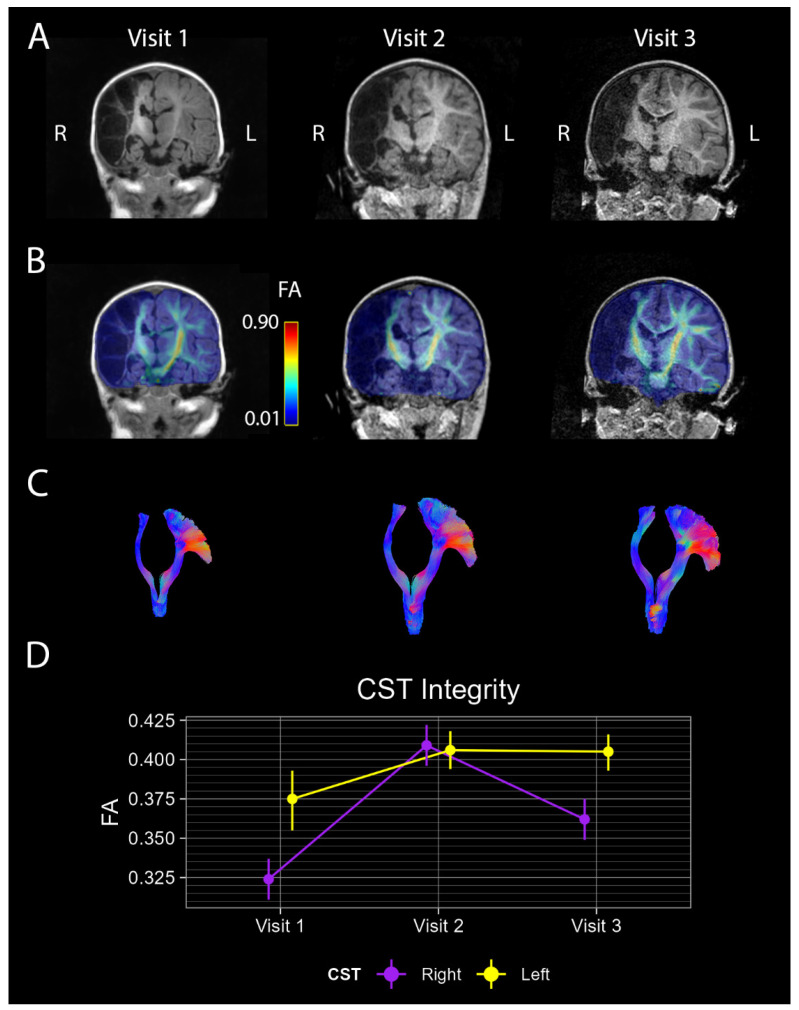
MRI characterization of brain injury and CST integrity across visits. (A). Coronal views of T1w images from Visit 1 (left), Visit 2 (middle), and Visit 3 (right). The majority of the right hemisphere has been replaced by cerebrospinal fluid following perinatal ischemic stroke. (B). FA maps derived from processed dMRI scans overlaid over T1w images from (A). CSTs are evidenced in left and right hemispheres as warmer colored regions between cortex and brainstem. (C). CST tract images generated by Tractseg. CSTs were identified in both hemispheres, matching with the higher FA regions shown in (B). Color mapping shows the orientation of the white matter bundles (red—left-right, green—anterior-posterior, blue—inferior-superior) (D). Quantification of FA values from right (purple) and left (yellow) CSTs at both study visits. Dots indicate the weighted-mean FA while lines indicate the bootstrapped 95% CI of the weighted mean.
